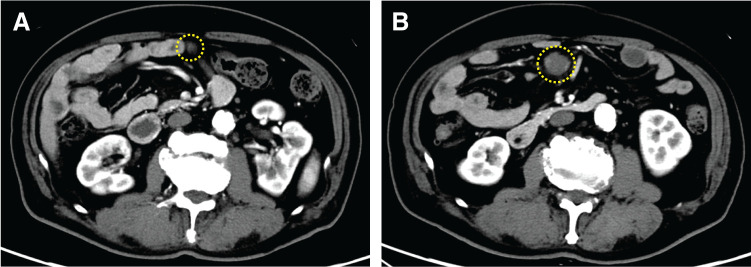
Abdominal CE-CT images taken 14 months prior (A) and at initial consultation (B). The mass lesion in the small intestinal mesentery showed contrast enhancement; it had become larger during this period (yellow circle outlines). CE-CT, contrast-enhanced-CT
